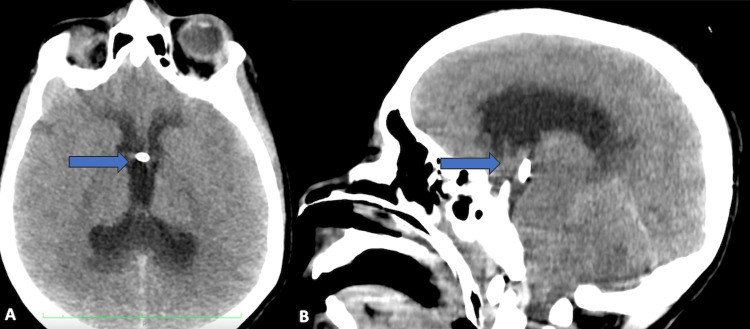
Non-contrast CT images of the head with axial (A) and sagittal (B) reconstructions showing the external ventricular drain (EVD).
